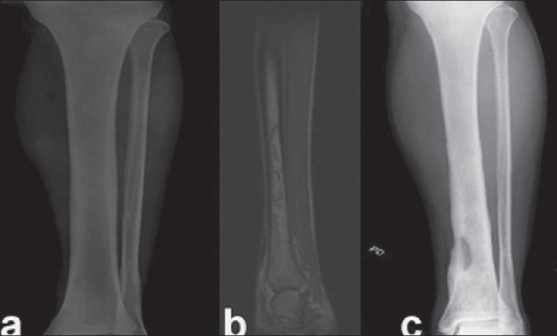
(a) X-ray anteroposterior view of left tibia showing normal appearance. (b) T2W MR image of left tibia showing intramedullary abscess. (c) X-ray anteroposterior view of left tibia shows healed osteomyelitis of lower end tibia after 22 months
